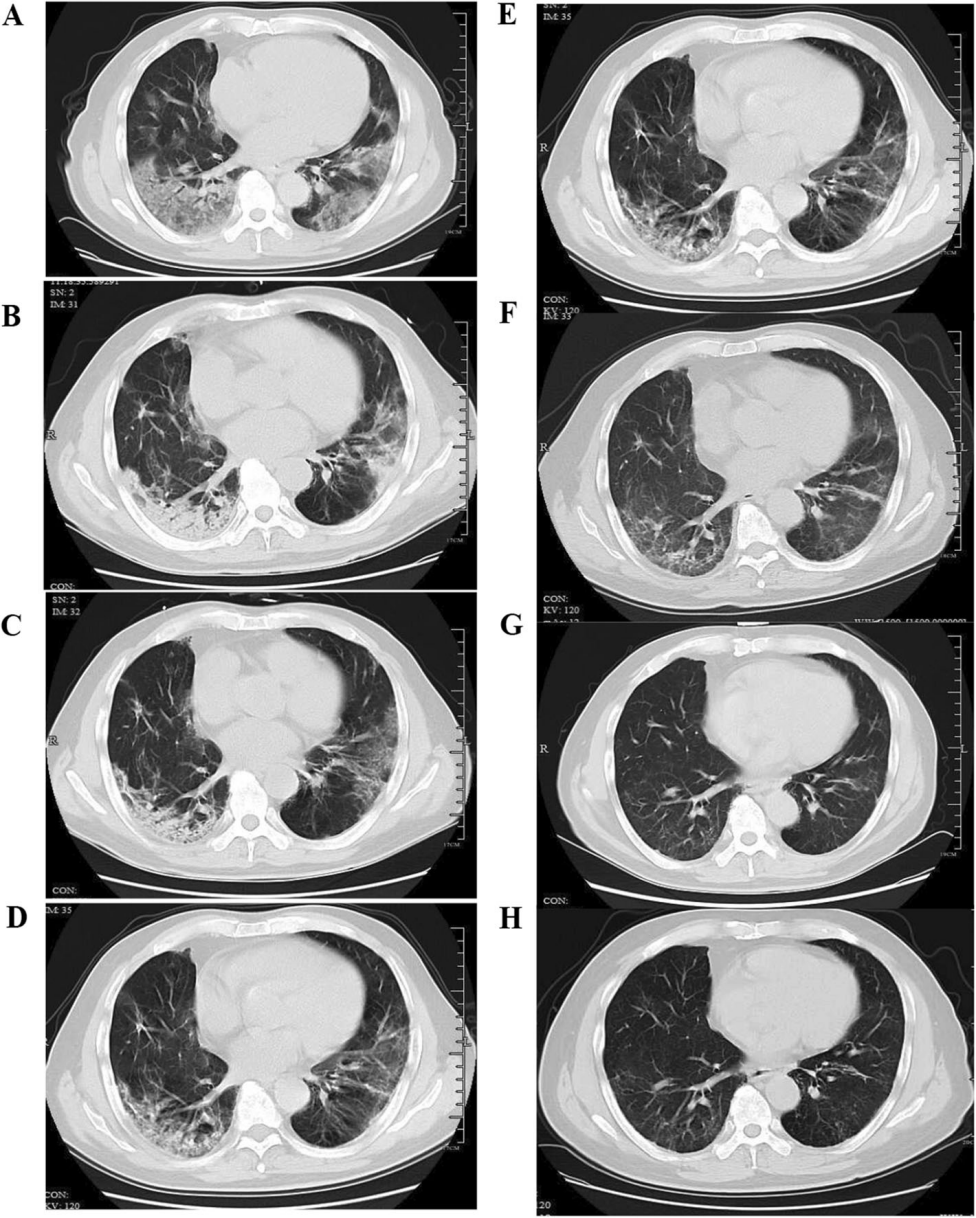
Transverse CT scans of a 72-year-old man with severe COVID-19. He was presented with fever and cough on January 29, 2020, confirmed with SARS-CoV-2 infection on February 1, then admitted to the hospital on the same day, and was treated with corticosteroid and high flow nasal cannula during hospitalization. A Scan obtained on day 7 showing diffuse subpleural GGO. B Scan obtained on day 14 showing subpleural GGO with partial consolidation. C Scan obtained on day 21 of illness showing subpleural GGO, and consolidation partly absorbed. D Scan obtained on day 28 of illness showing irregular linear opacities that developed in the areas of GGO. E Scan obtained on 1 month after discharge (day 62 of illness) demonstrating irregular linear opacities and subpleural reticulation. F Scan obtained on 3 months after discharge (day 112 of illness) showing subpleural reticulation. G Scan obtained at 6 months after discharge (day 210 of illness) showing slight irregular linear opacities and reticulation on the basis of GGO. H Scan obtained at 1 year after discharge (day 390 of illness) showing subtle irregular linear opacities and reticulation on the basis of GGO
